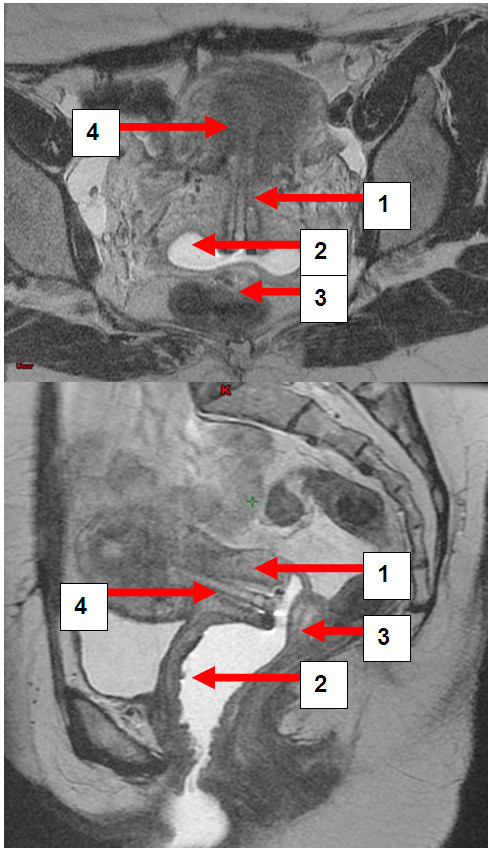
a and b: Patient BW. Transversal and sagittal pelvic MRI (T2-FSE) with cervical carcinoma (1) vaginal gel (2) and rectal separation (3).
